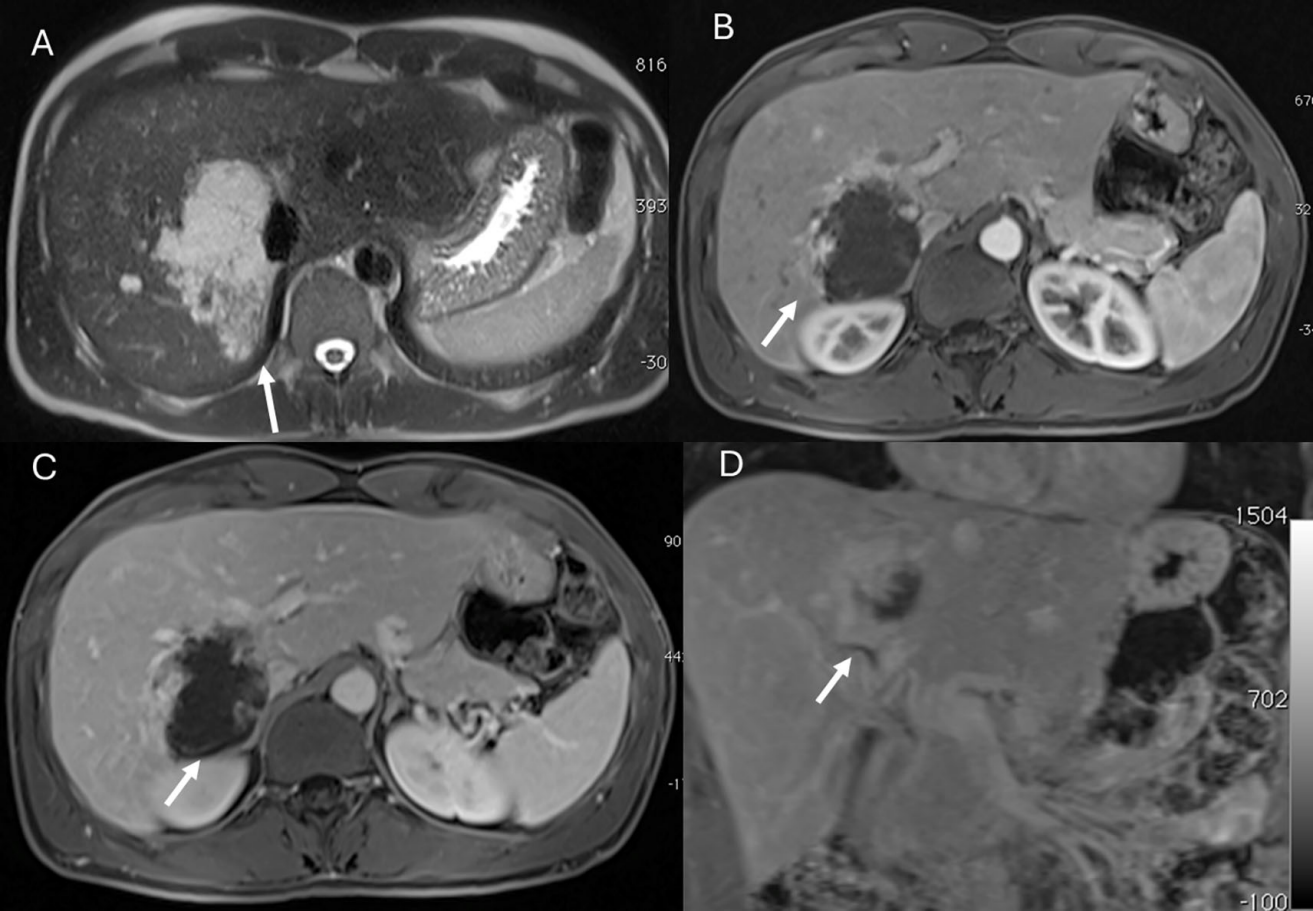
MRI assessment of liver hemangiomas (arrows). In (A) (T2-W sequence, axial plane), the hemangioma (arrow) shows hyperintense signal, and after non-specific contrast agent administration a progressive enhancement is seen (B) T1-W in axial plane during in arterial phase; (C) T1-W in axial plane during portal phase and (D) T1-W in coronal plane during late phase).
